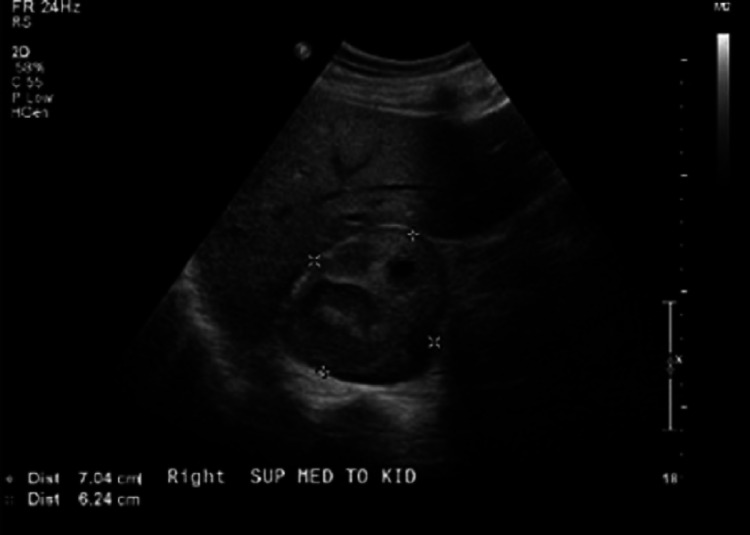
Retroperitoneal ultrasound showing 7.0 x 6.2 x 7 cm heterogenic mass in right adrenal gland
